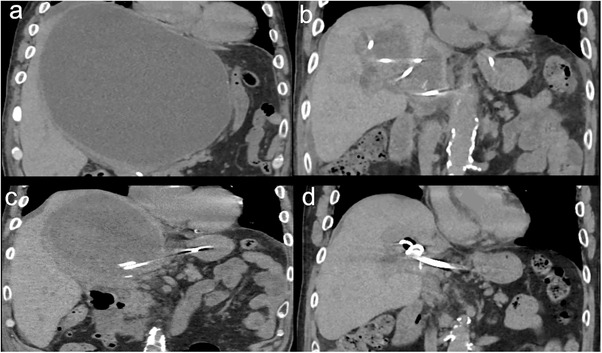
Sequential computed tomography (CT) findings of the infected hepatic cyst. (a) On readmission, the giant hepatic cyst shows wall thickening and internal hyperdensity, consistent with infection. (b) After external drainage, the cyst collapsed, increasing the distance between the cyst and the gastric wall. (c) Twenty‐two days after internal stenting, recurrence occurred due to stent occlusion. (d) Six months after placement of three internal plastic stents, the cyst remained collapsed without recurrence.
